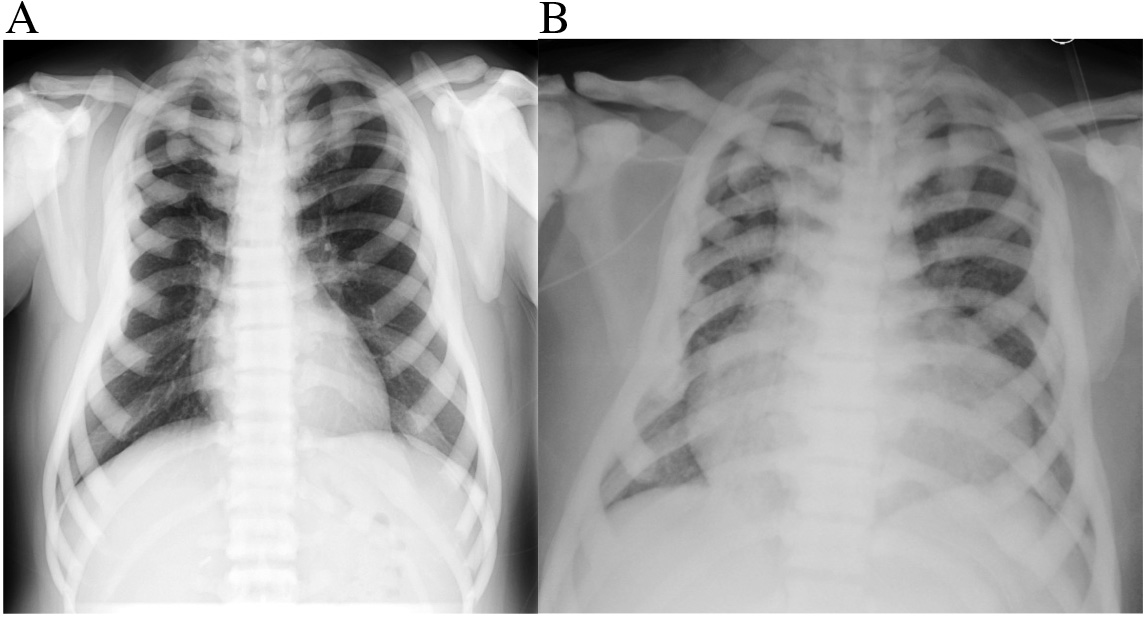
Chest radiographs before and after transplantation in adult with osteopetrosis. Chest radiographs are shown for patient 2, (*A*) before transplantation and (*B*) after transplantation, demonstrating generalized increased bone density of all bones, and a rugger Jersey spine appearance.
